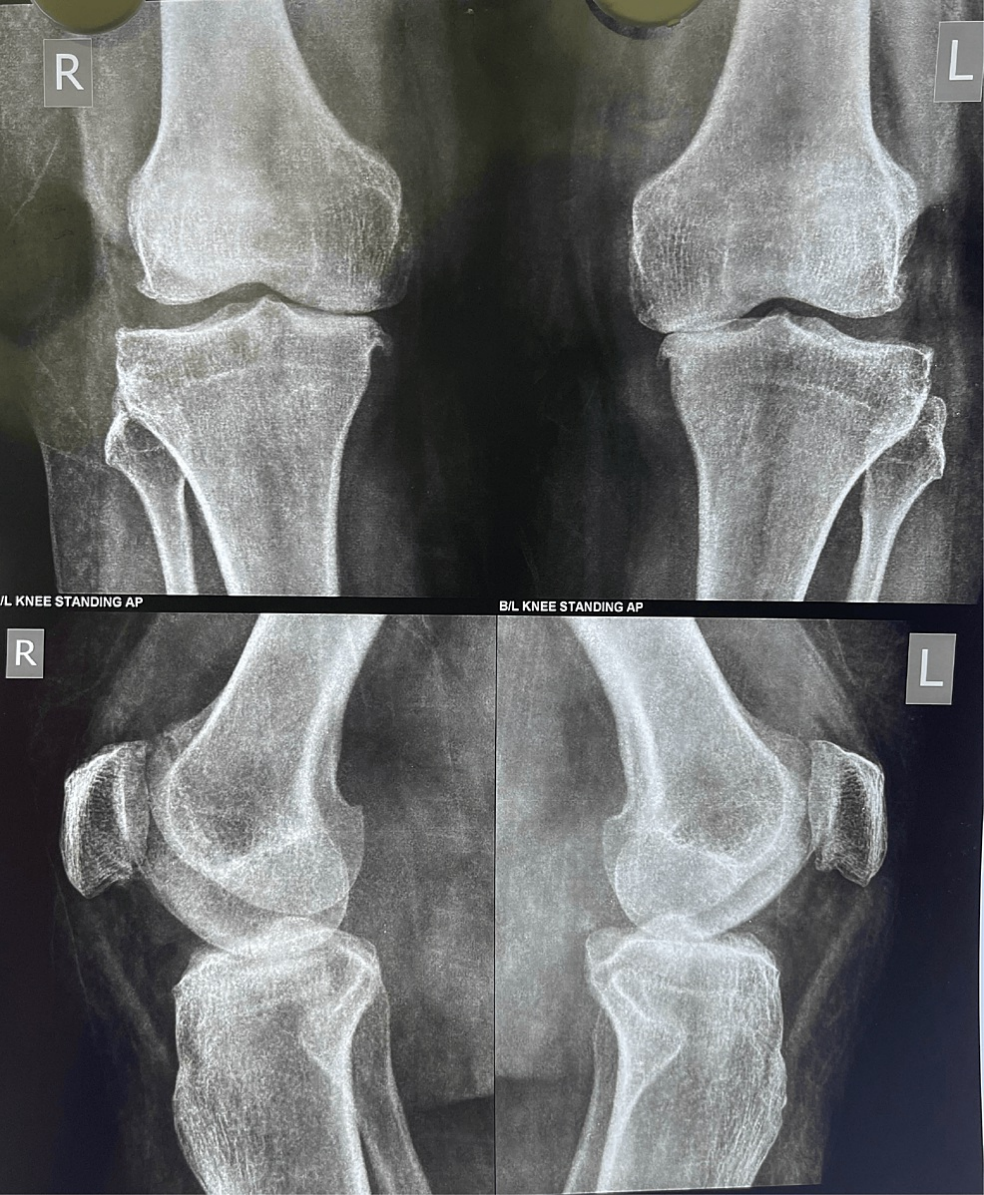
Preoperative radiograph showing a varus deformity with severe osteoarthritis over the medial side in both knees
